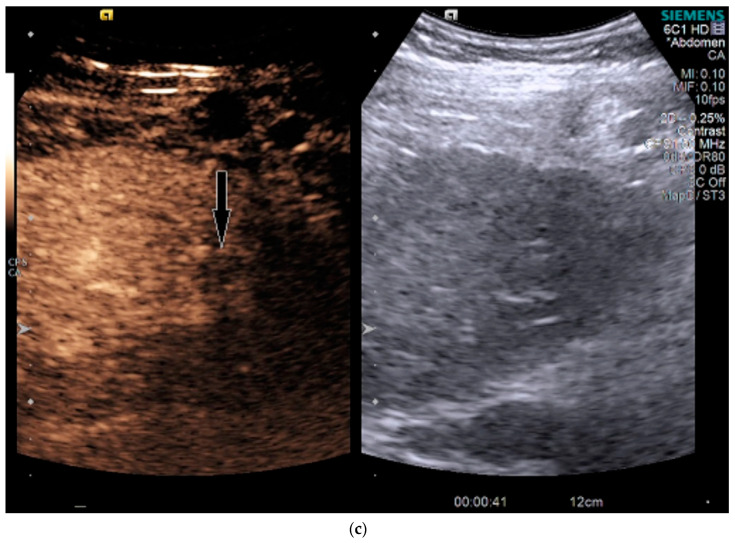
Patient 2 ultrasound images, with liver lesion denoted by black arrow: (a) grayscale images showing the hypoechoic lesion. Post contrast administration ultrasound in arterial phase (b) and early washout (c) of the lesion, LI-RADS M.
